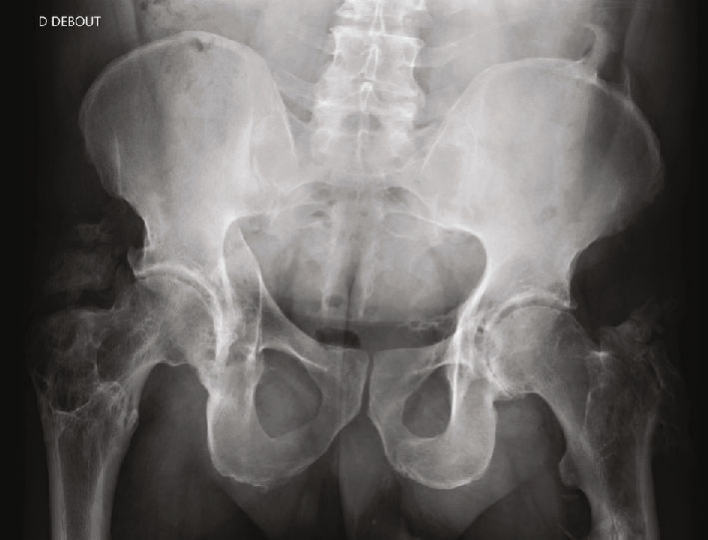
Follow-up X-ray over 20 years postsurgery (AP view).
